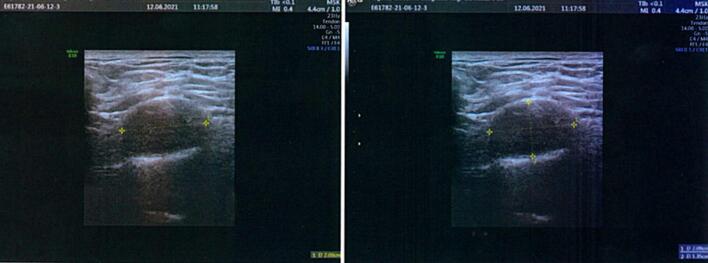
Ecography of the brachial plexus area, showing the presence of a Schwannoma-compatible nodule, suggesting further investigation by MRI.
